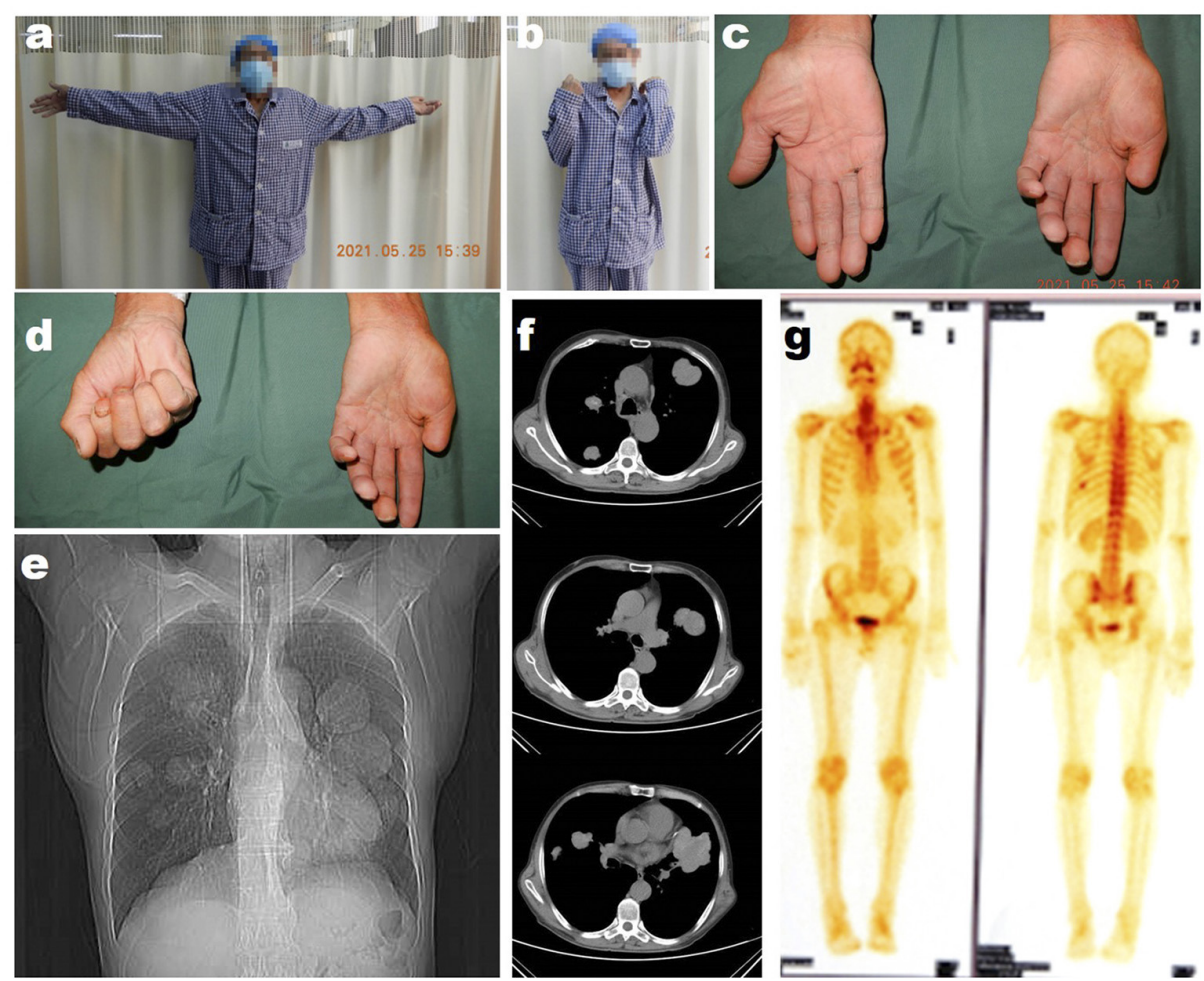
The preoperative examination of the patient's second operation in our hospital. (a) The photograph of the patient trying to abduct his bilateral shoulders. (b) The photograph of the patient trying to flex his bilateral elbows. (c) The photograph of the patient trying to extend his bilateral fingers. (d) The photograph of the patient trying to flex his bilateral fingers. (e) The radiological image of the patient's chest. (f) The representative CT images of the patient's chest. (g) The bone scan images of the patient.
